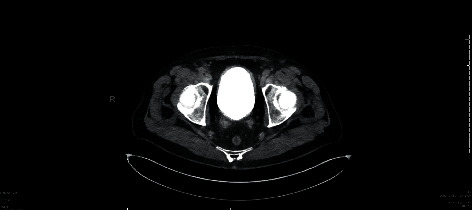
CT cystogram image displaying a contrast filled bladder. No extravasation/leakage of contrast from the bladder was noted.
